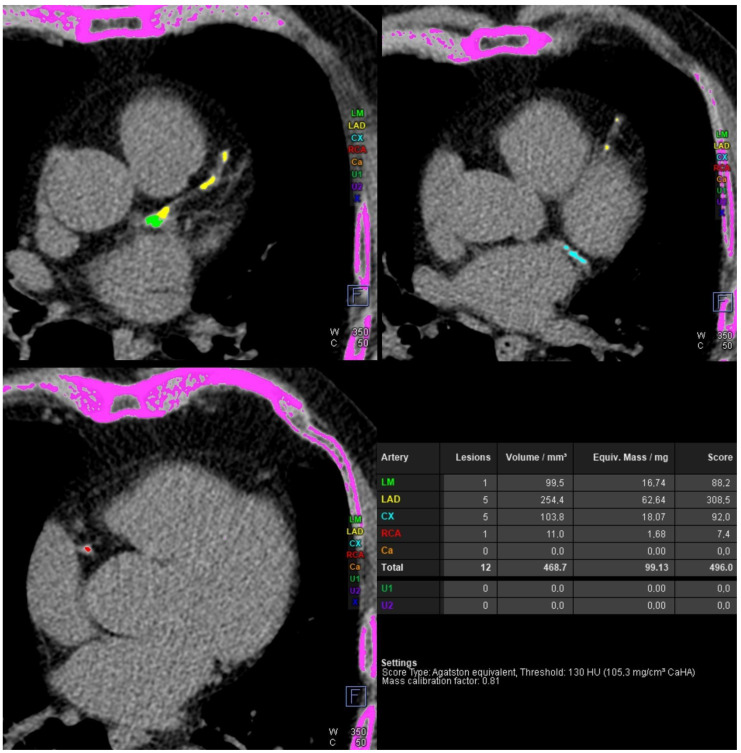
Coronary computed tomography angiography. Native phase. Coronary artery calcium score assessment. Calcifications in the left main (LM) are marked in green, the left anterior descending (LAD) in yellow, in the left circumflex (LCx) in blue, in the right coronary artery (RCA) in red and extracoronary calcifications in magenta.
